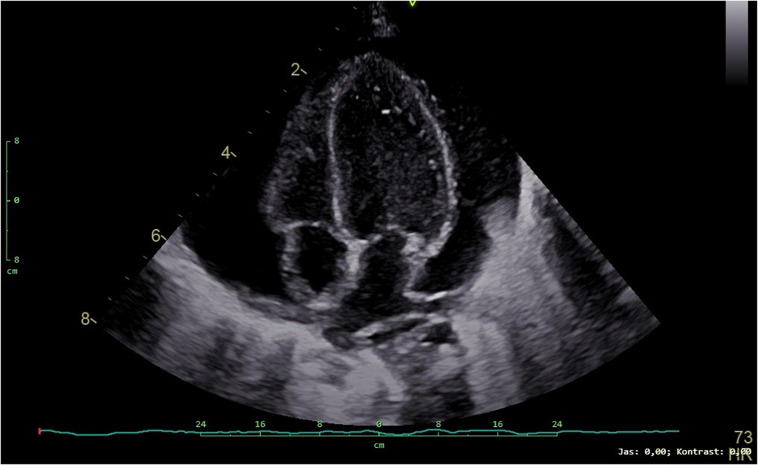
Transthoracic echocardiogram (4-chamber view) showing a large pericardial effusion in our patient.
